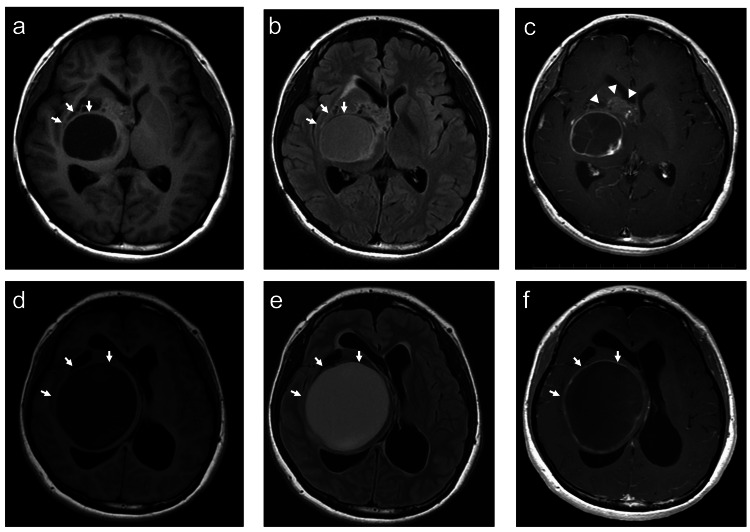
Response to chemotherapy and subsequent progression (a,b) After chemotherapy, MRI revealed hypointensity on T1-WI and isointensity on fluid-attenuated inversion recovery (FLAIR) within the cystic component (arrows). (c) The contrast-enhancing solid tumor component shows interval regression on contrast-enhanced T1-WI (arrowheads). (d,e) Follow-up MRI obtained two years after chemotherapy demonstrates progressive enlargement of the cystic component, with hypointensity on T1-WI and hyperintensity on FLAIR (arrows). (f) Contrast-enhanced T1-WI revealed enhancement of the cyst wall (arrows).
